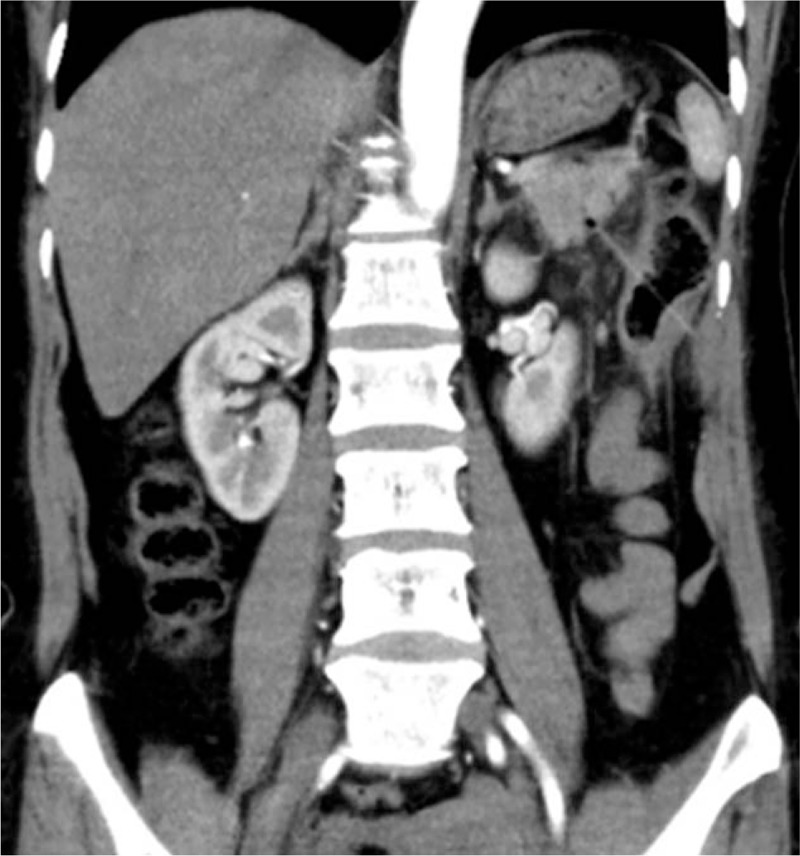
Coronal CT images show the continuous high-density point which can preliminarily tell us that a foreign body penetrates the wall of the intestine. CT = computed tomography.
